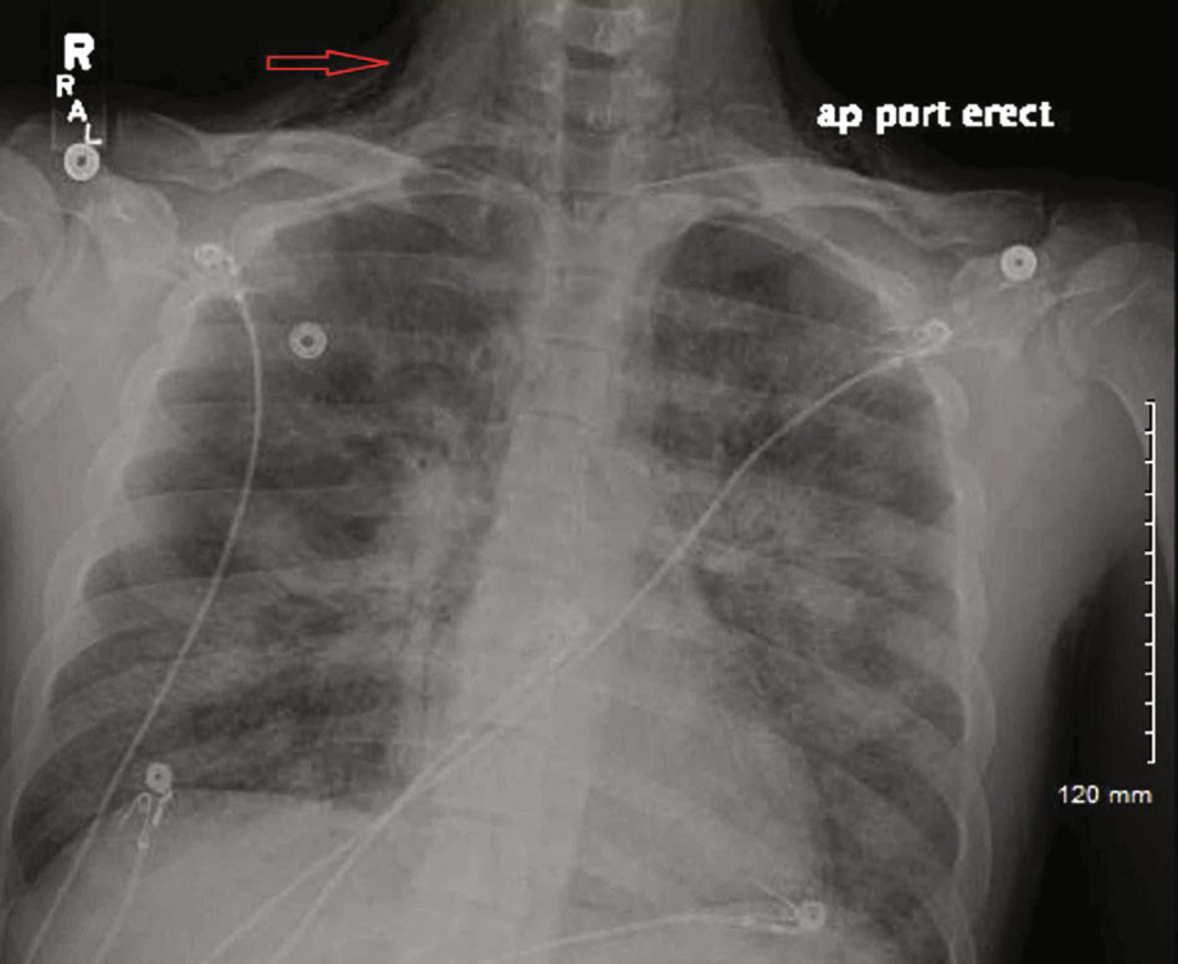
X-ray showing pneumomediastinum with subcutaneous emphysema (red arrow) extending to the cervical region with bilateral pneumonia.
